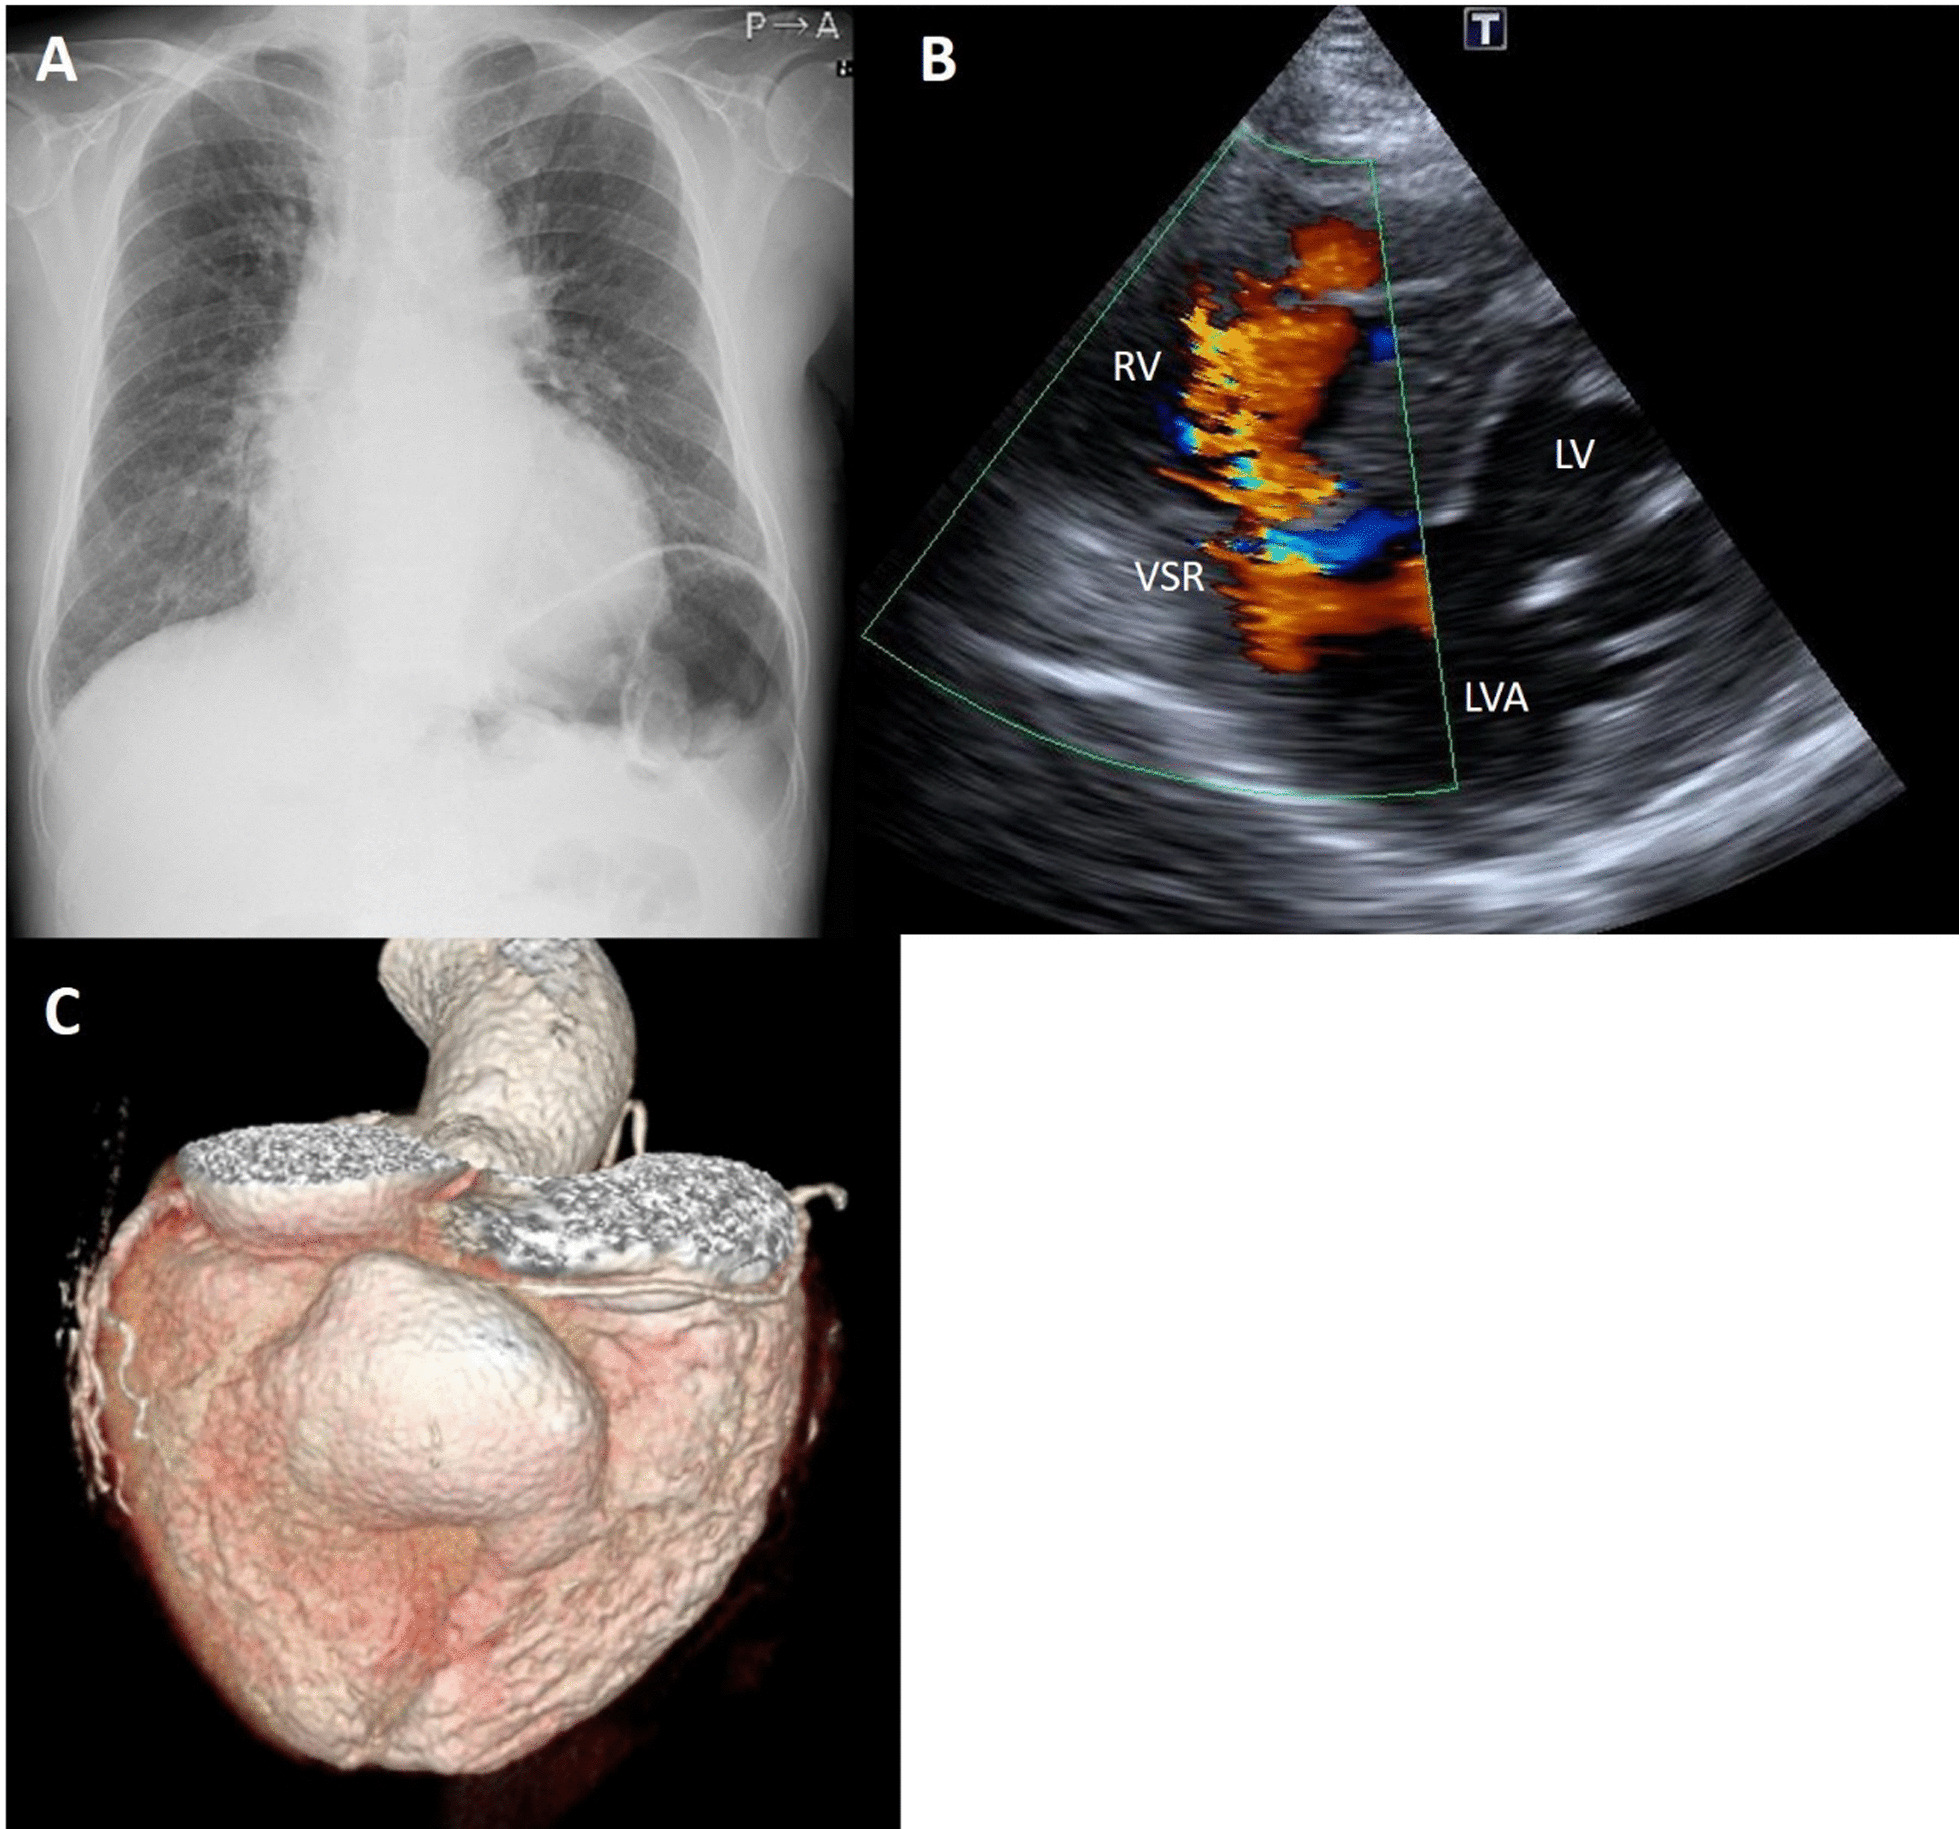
a Chest radiograph showed cardiomegaly and pulmonary edema. b Echocardiography at 10 month after presentation detected left-to-right shunt. c Three dimensional volume rendering image of cardiac computed tomography revealed an inferior LV aneurysm. *LV* left ventricle, *RV* right ventricle, *LVA* left ventricular aneurysm, *VSR* ventricular septal rupture
